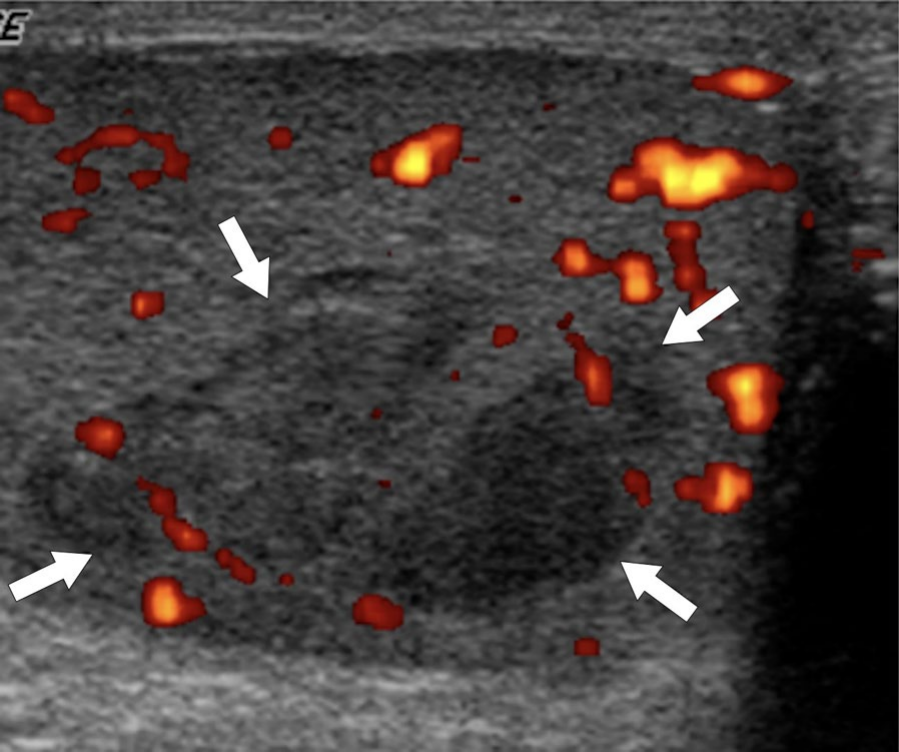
Isolated testicular vasculitis. A 35-year-old healthy male with progressive severe testicular pain and palpable mass on physical exam. Power Doppler ultrasound image demonstrates a hypoechoic peripherally located testicular mass (arrows) with mild increase in perilesional blood flow. Orchiectomy revealed necrotizing vasculitis and hemorrhagic infarct. There was no evidence of systemic vasculitis elsewhere in the body in the post-operative medical evaluation
